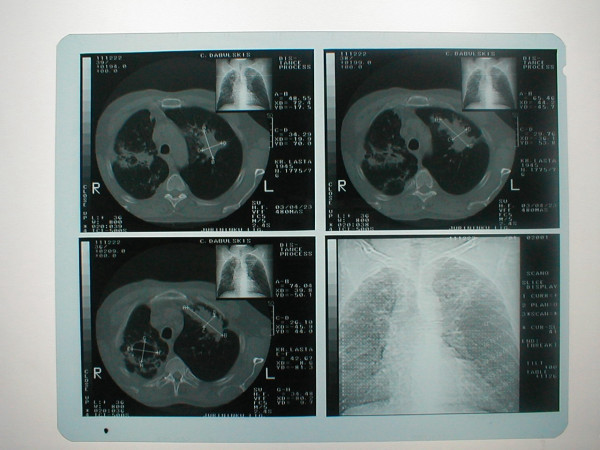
Chest CT. There is pulmonary bulla in the right S1 segment, and focus of mottled opacity 7,5 × 2,5 cm with infiltration around it.
